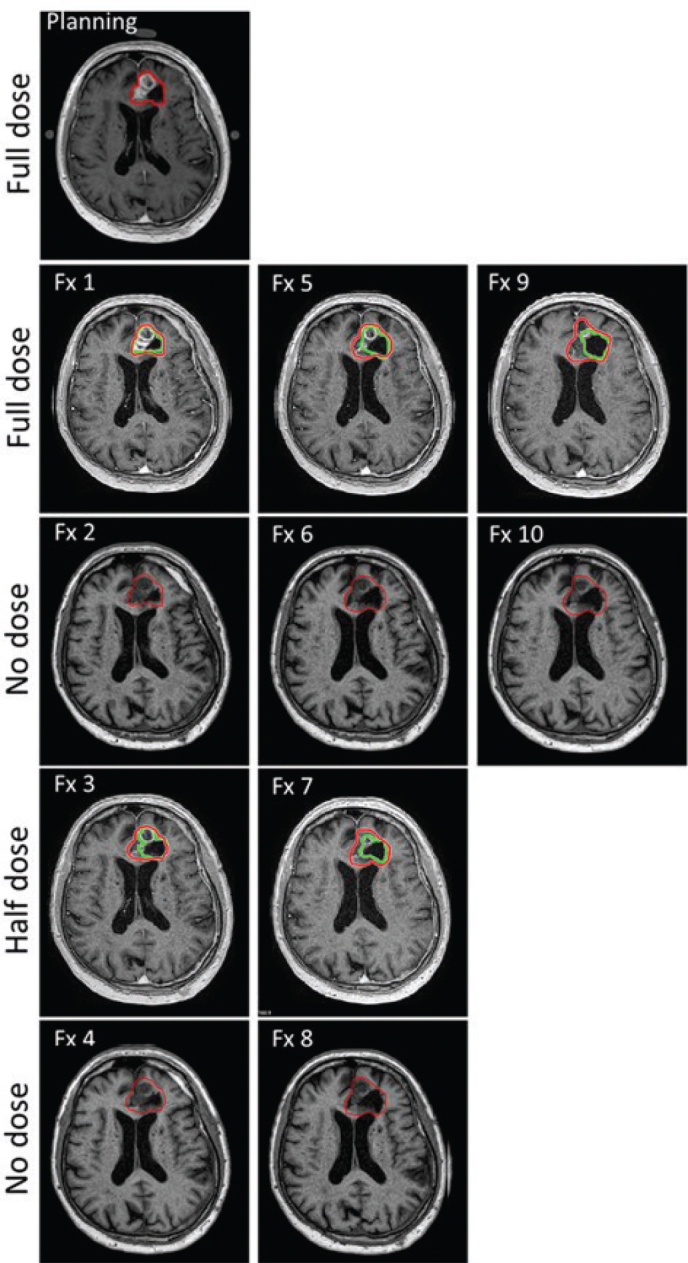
T1W magnetic resonance imaging (MRI) scans of patient 8 with decreasing tumour volume during the radiotherapy course. The online delineations (Fx 1 to Fx 10) are in red remaining relatively constant over time and the offline expert delineation are in green showing decreasing GTV volume. Fraction 1 was delivered 7 days after the planning MRI.
